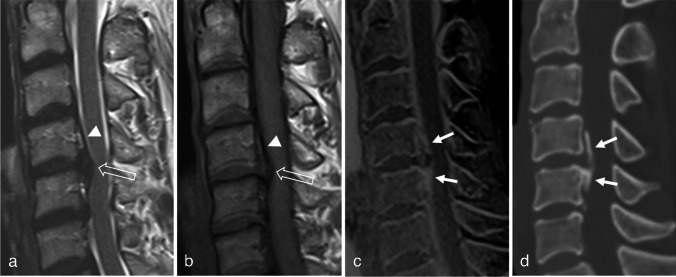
Fifty-three-year-old female who presented with posterior neck pain and radiating pain to right upper extremity. Sagittal T2-WI (a) and T1-WI (b) show disk herniation (open arrows) at C4/5 level. The 3D FLASH image (c) revealed the OPLL at C4,5 level (arrows). The sagittal CT image (d) confirms the diagnosis of OPLL (arrows). At the first reading session with sagittal TSE images, both readers could not diagnose the OPLL. The elevated thin hypointense structure (arrowheads in a and b) was thought to be the elevated posterior longitudinal ligament due to the herniated disk. After additional review of 3D FLASH image, both readers detected OPLL and corrected their decision
